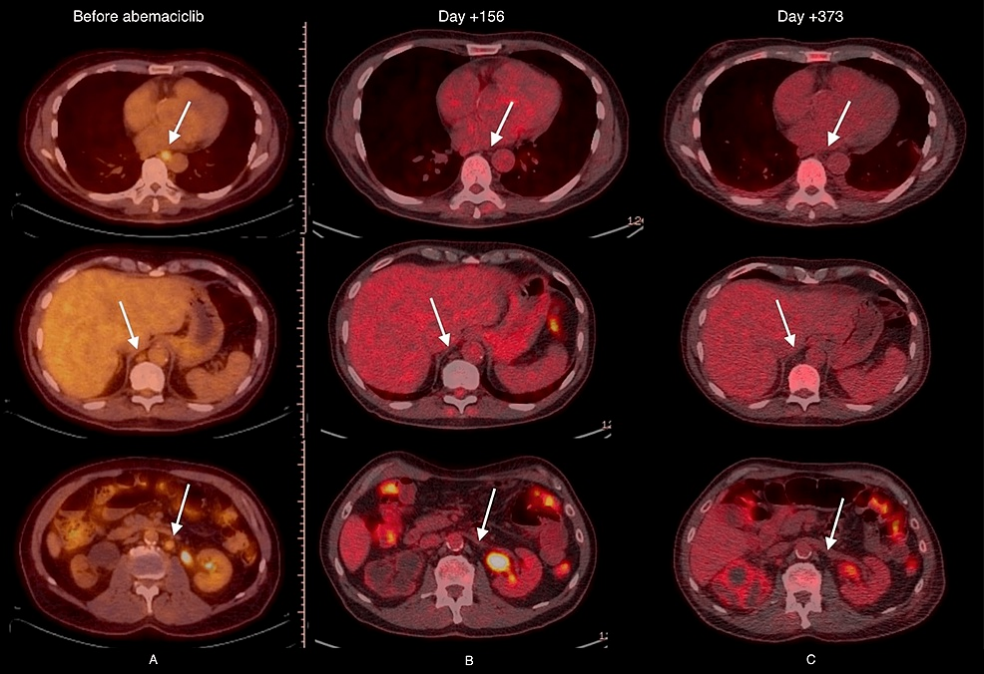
A) FDG-PET imaging obtained prior to administration of abemaciclib; B) imaging at day +156, indicating response; C) imaging at day +373, indicating durability of response.
